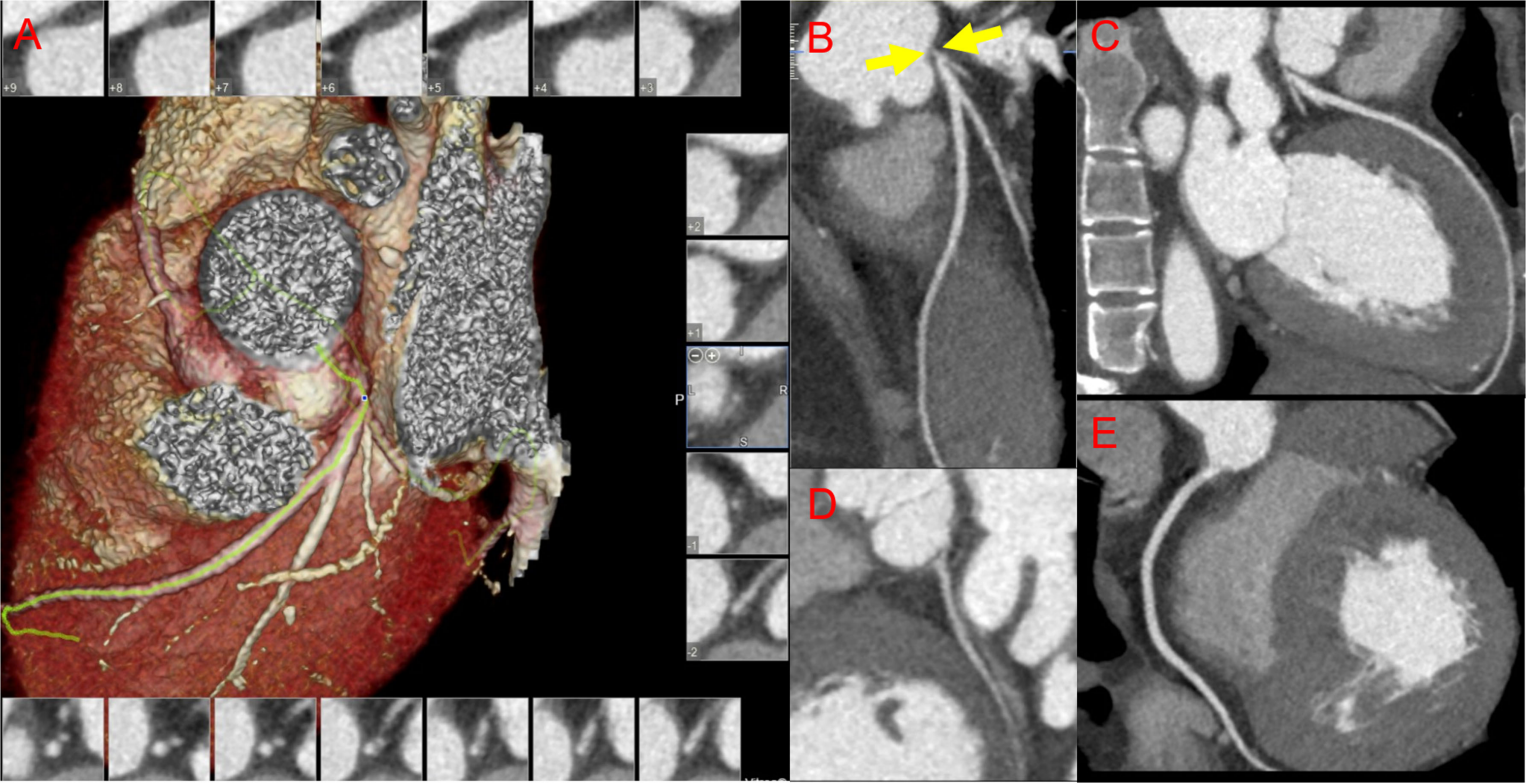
Images for coronary involvement in Takayasu arteritis. A 24-year-old woman with newly diagnosed Takayasu arteritis complicated with old myocardial infarction and heart failure presented with dyspnea for 1 year and had an monocyte-to-high-density lipoprotein ratio of 0.66. (A) Reconstructed imaging of coronary computed tomography angiography (CCTA); CCTA demonstrates severe stenosis in the left main coronary artery (B) (yellow arrows), and normal vessels of the left anterior descending coronary artery (C), the left circumflex coronary artery (D), and the right coronary artery (E).
